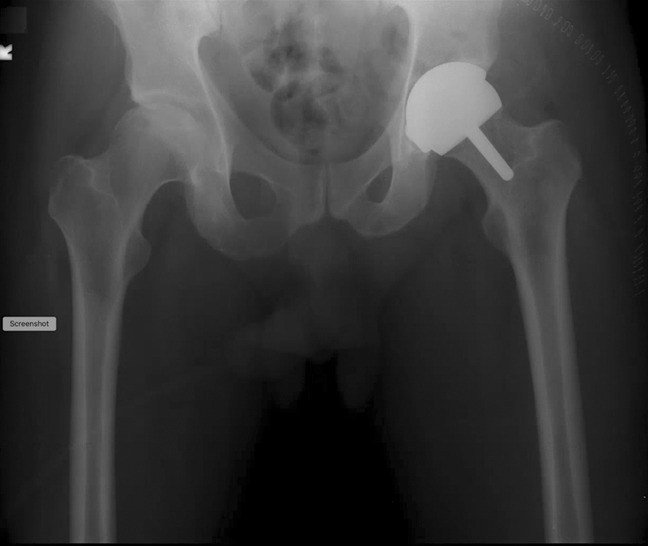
Postoperative radiograph after left Birmingham hip resurfacing procedure.
